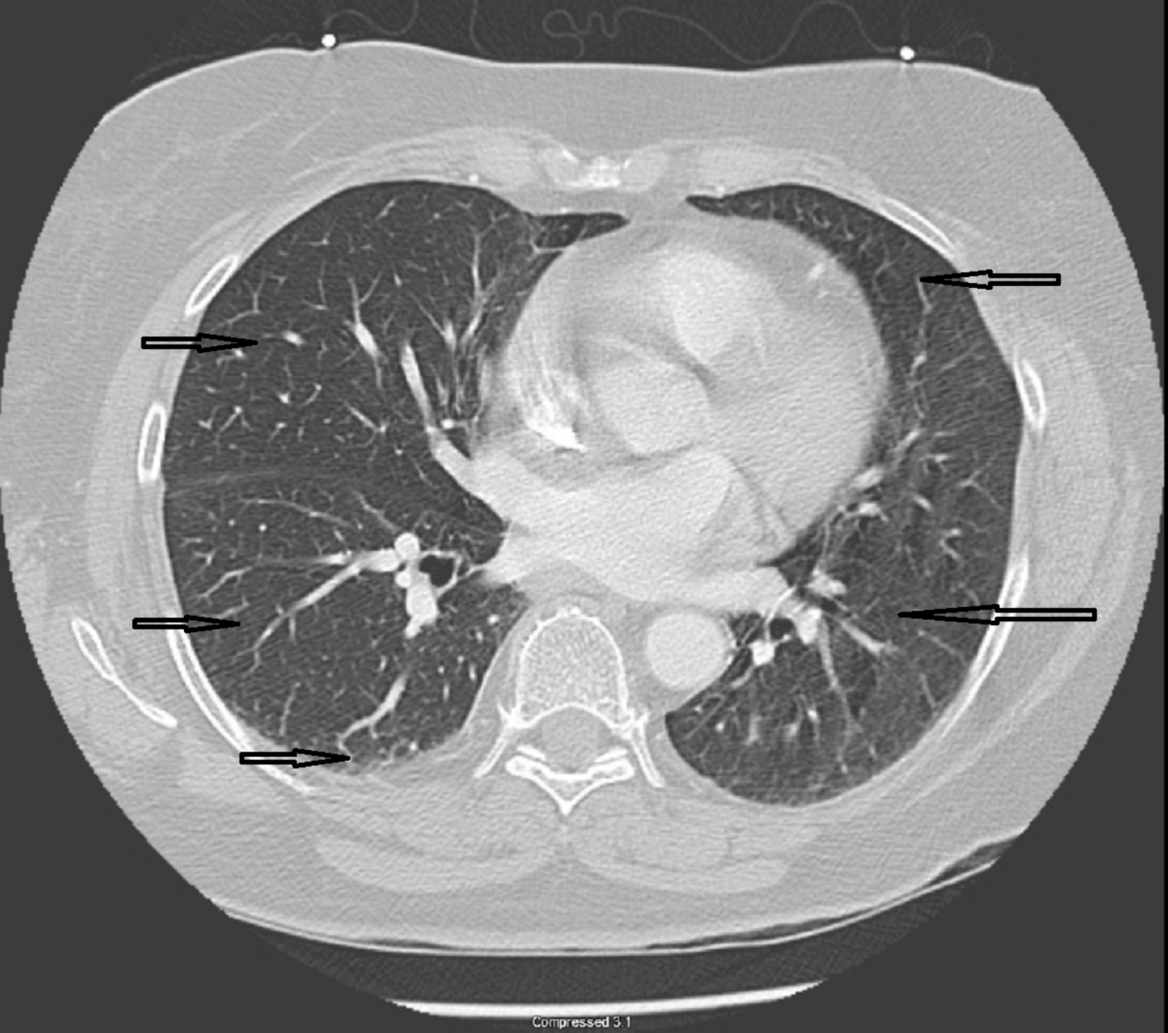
Computed tomography scan of chest showed emphysematous changes with no pulmonary embolism or aortic dissection (arrows pointing)
